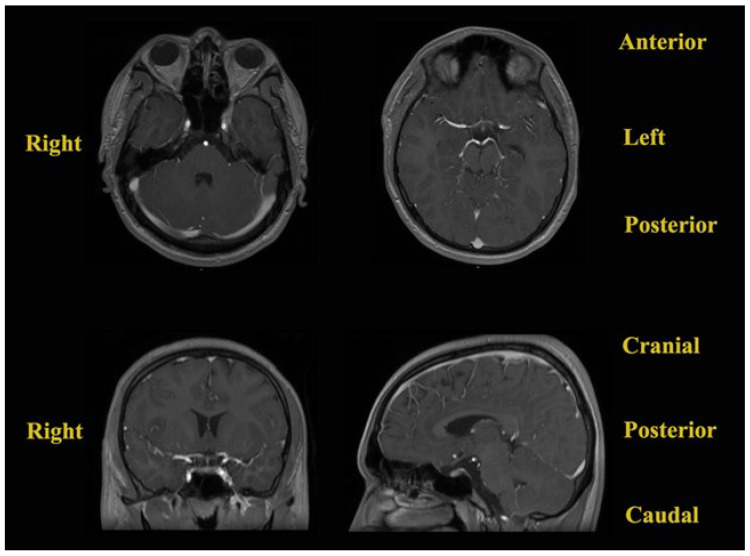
Contrast-enhanced brain MRI (T1-weighted sequences) performed at the 3-month follow-up.
